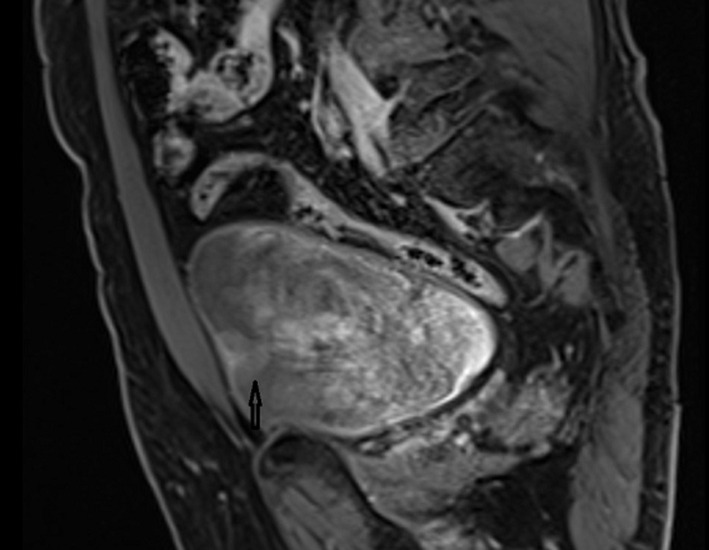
contrast enhancement in the polypoid lesion located in the bladder by magnetic resonance imaging
